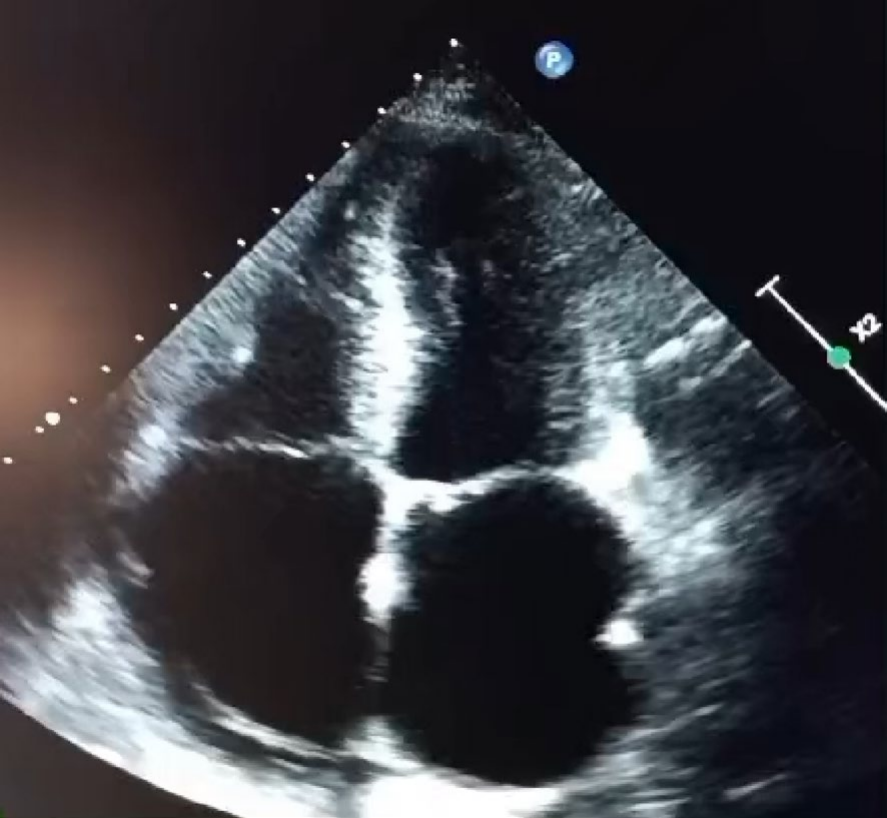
Apical four-chamber view showing concentric LV hypertrophy with granular myocardial texture and biatrial enlargement
